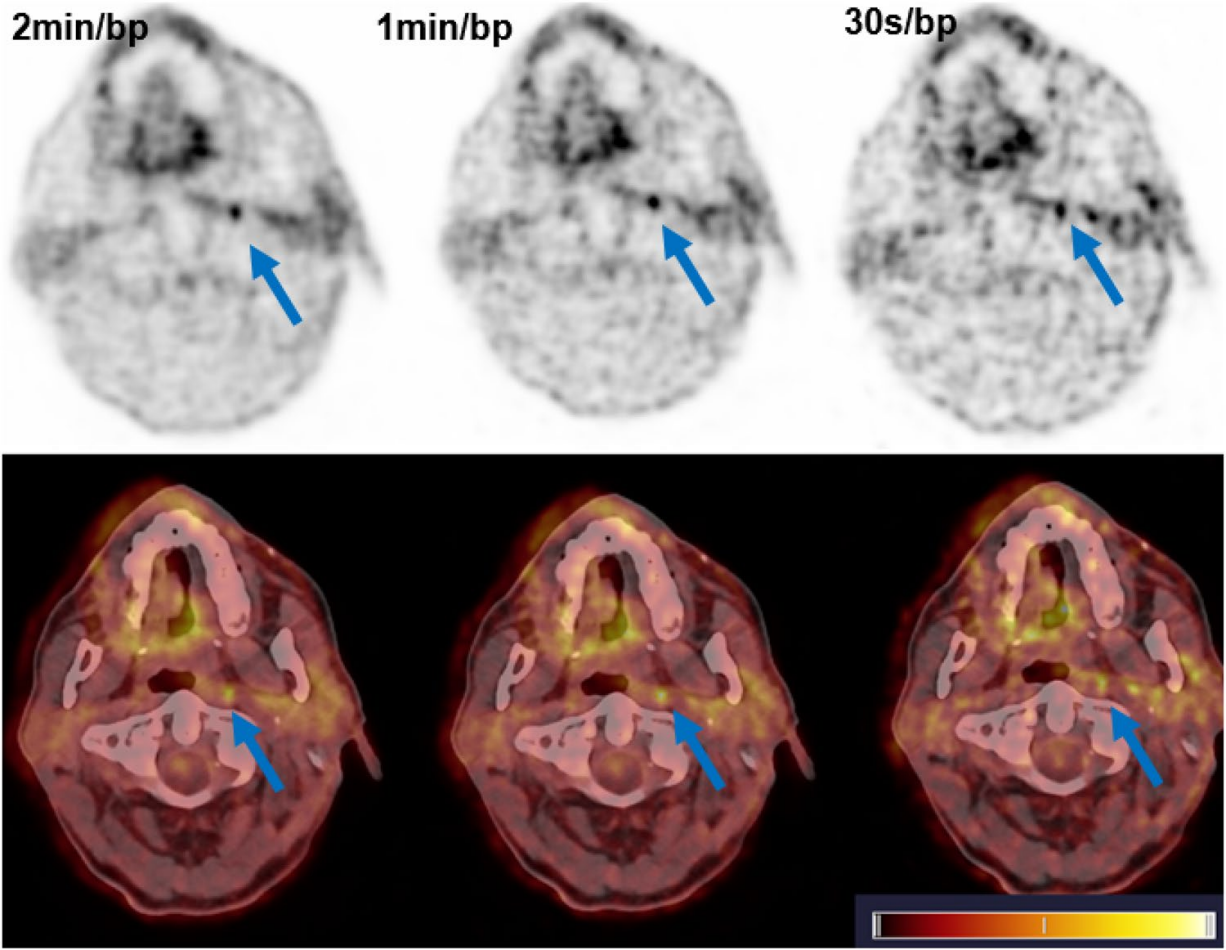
Example of lesion missed at shorter acquisition (30 s/bp) but easily identified at 1 min and 2 min/bp list-mode acquisition. This retropharyngeal lymph node (blue arrow) was overlooked owing to increased noise in the 30 s/bp acquisitions, and is much better delineated at 2 min and 1 min/bp. The top row represents the PET data, the bottom row fusion of PET and CT. Bottom right shows colour look-up table (scale 0–8 SUV)
